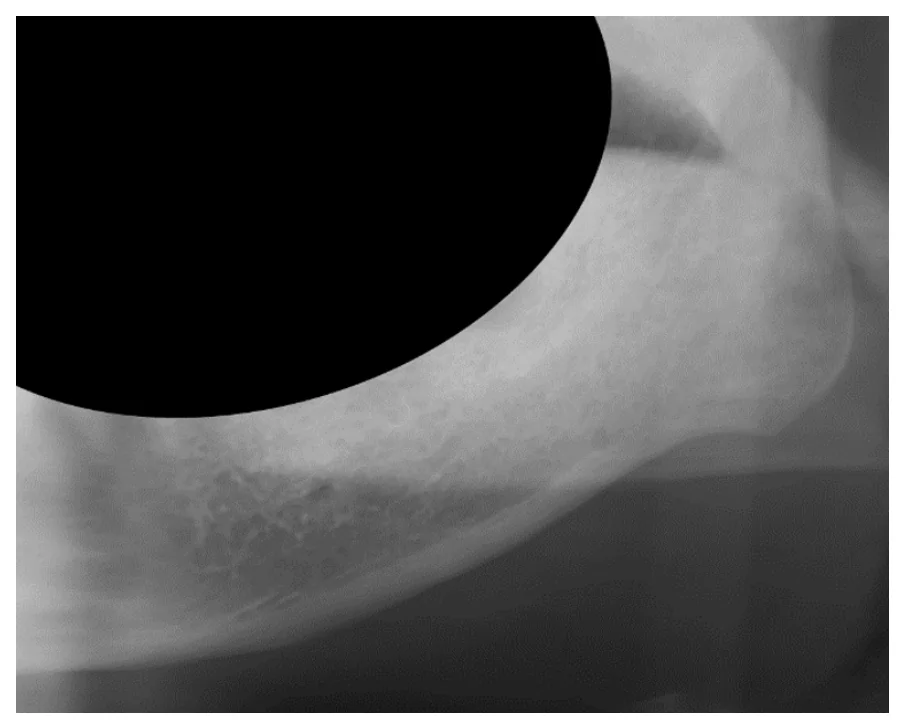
Cropped and masked panoramic radiograph prepared for MCI evaluation. To protect patient privacy, the image was trimmed at the midline to display only the left mandibular inferior cortex, and the dentition region was obscured with a black oval overlay to prevent age-related diagnostic bias. Patient identification data were removed, and images were stored as JPEG files without DICOM annotation.
